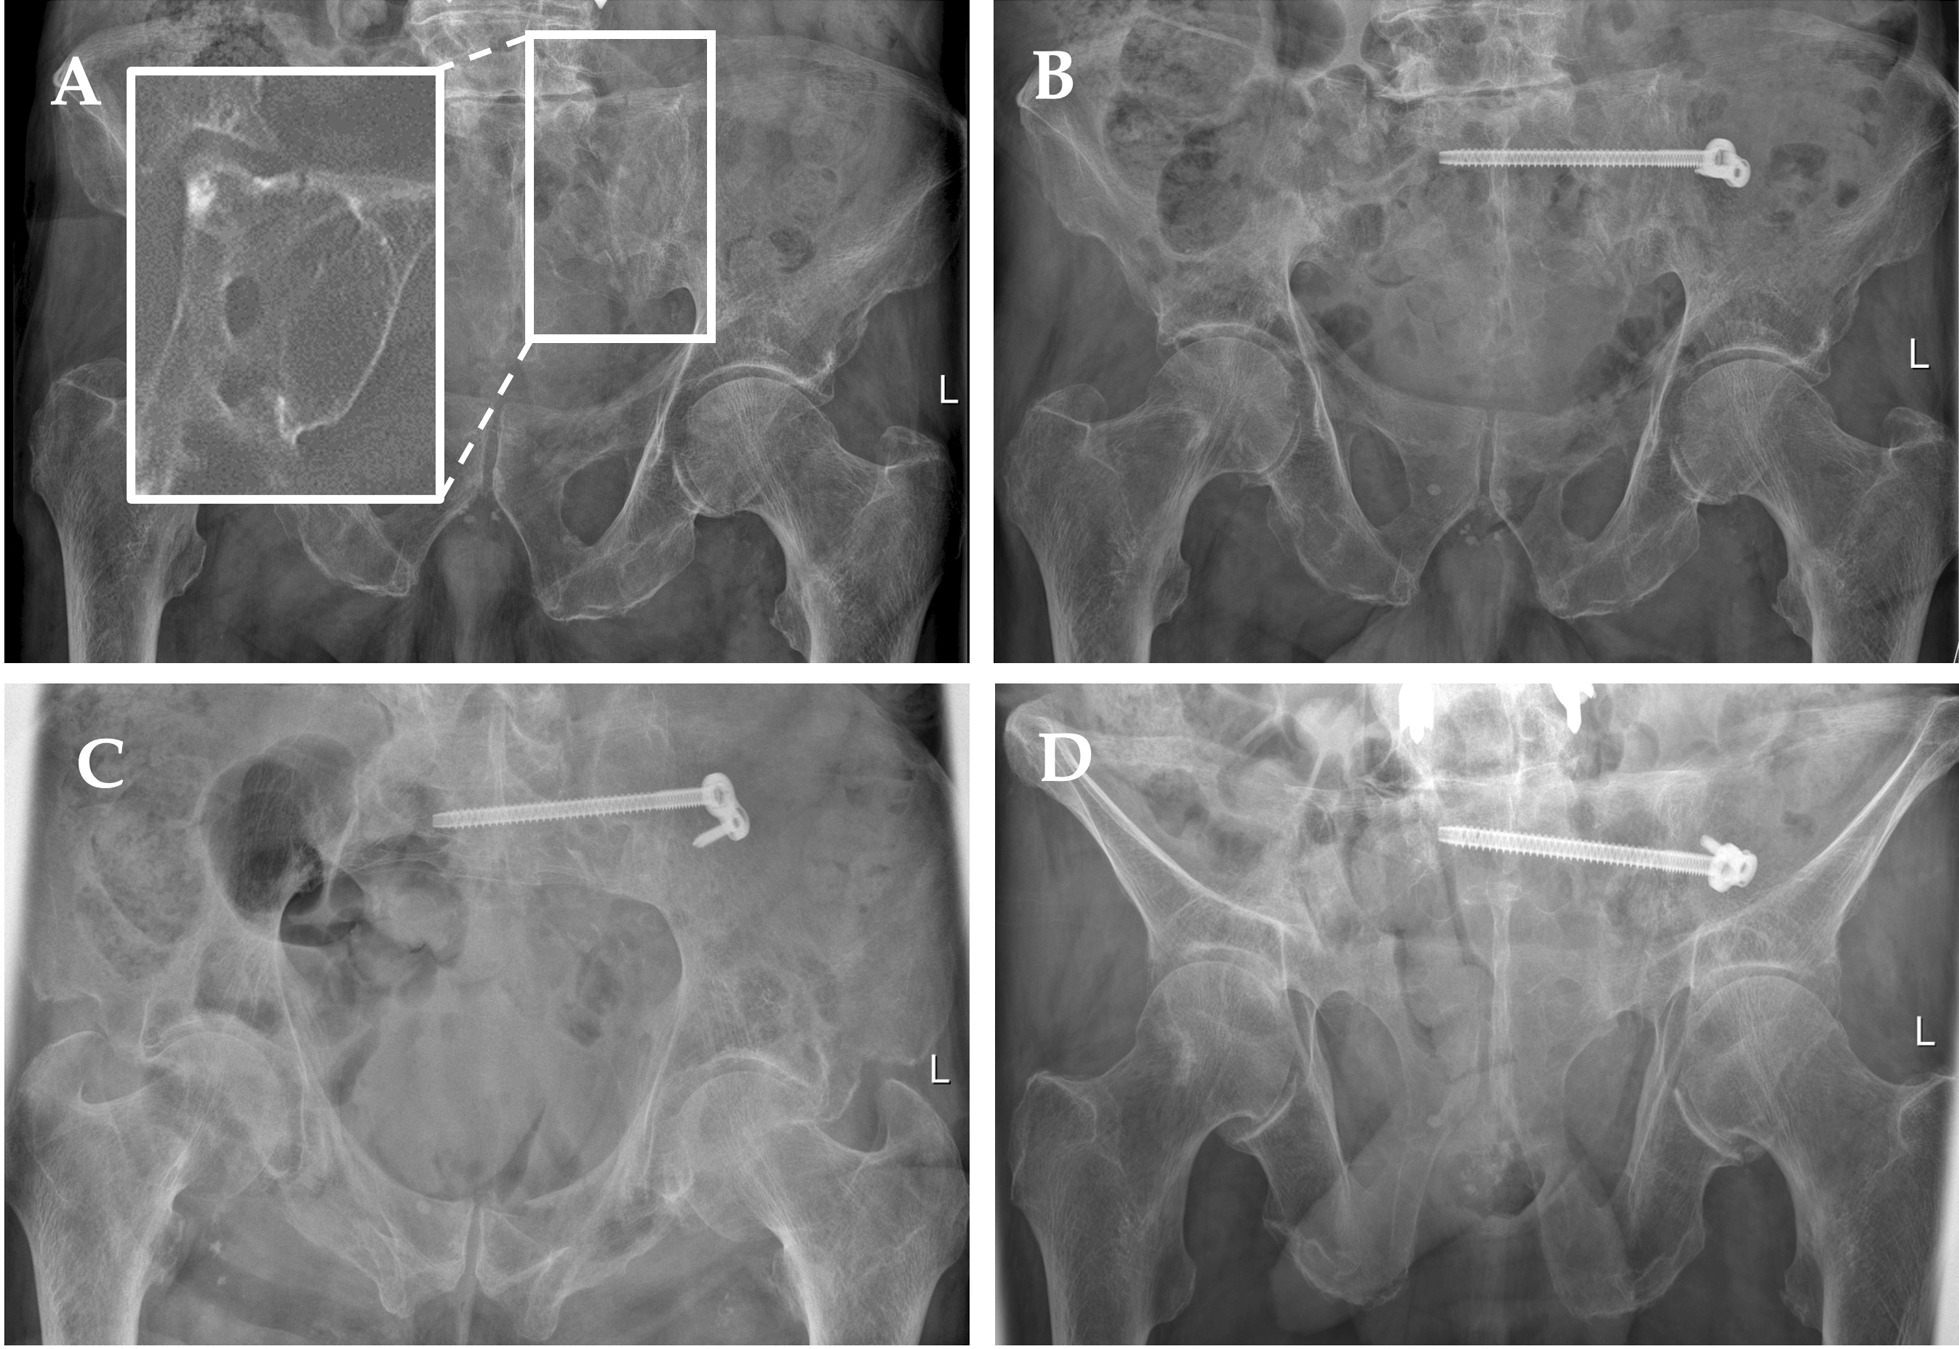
Case of a FFP type IIb fracture in an 81-year-old male patient after a fall from a standing position. a Initial x-ray of the pelvis in anterior–posterior view with a magnification of the left sacrum from the CT-scan. b Post-operative x-ray control in anterior–posterior direction after fracture stabilization using the iliosacral screw with washer plate and angular stable ilium screw. c Inlet-view. d Outlet-view
